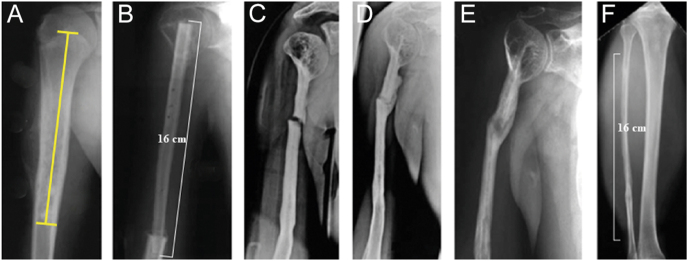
Sixteen-year-old boy with chondrosarcoma of the right humerus; (A) preoperative anteroposterior radiograph; and (B) postoperative radiograph after further resection (R0) and implantation of a non-vascularized fibula graft. The fibula was wedged only in the proximal and distal parts of the humerus; (C) traumatic transverse fracture of the graft 6 years after reconstruction. Conservative treatment consisted of an upper arm brace; (D) good callus formation after 3 months; (E) 26.5 years after the operation with integration and remodeling of the transplant; (F) complete regeneration of the fibula.
